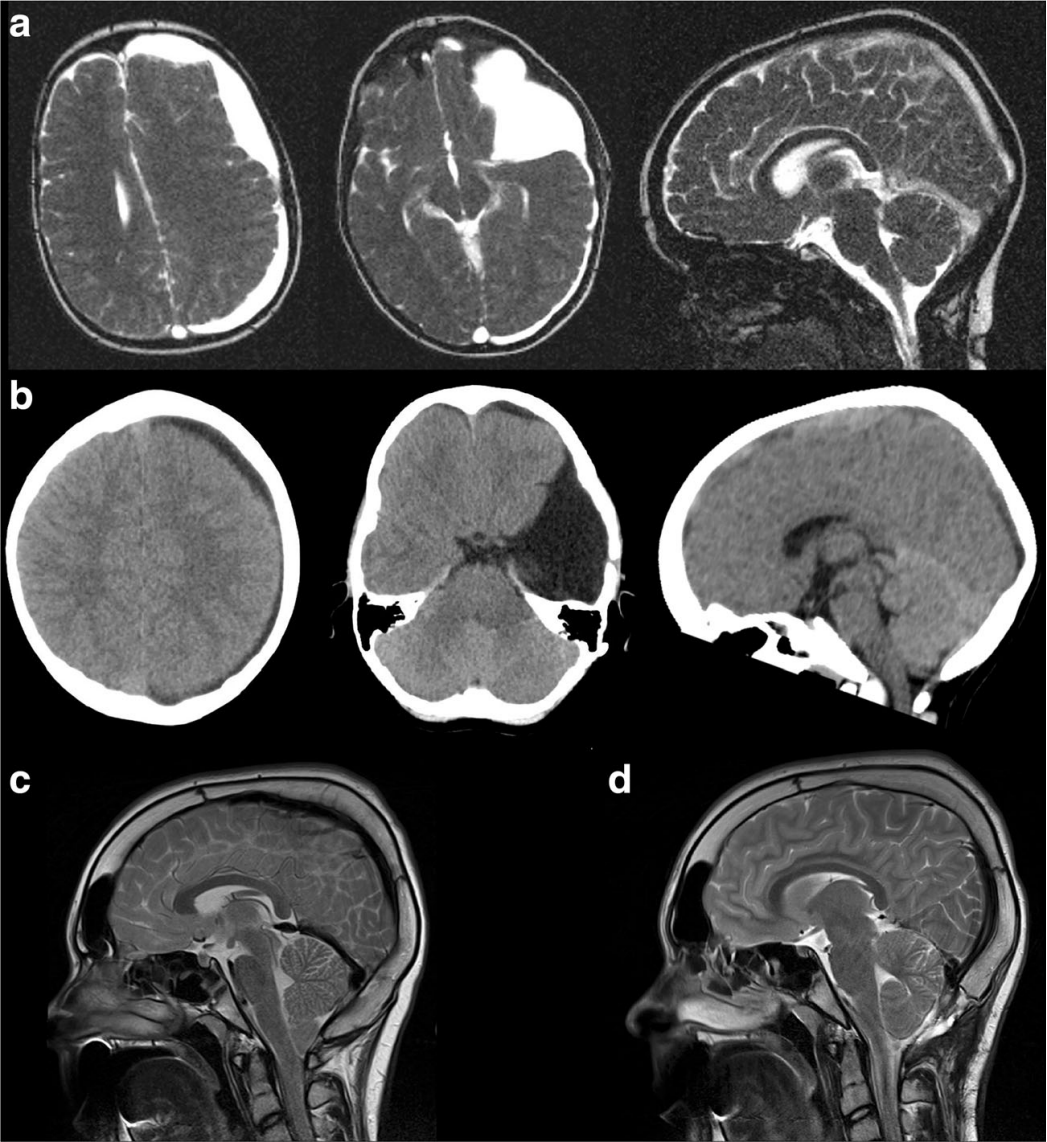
CT and MRI scans of a 3-year-old boy with a bleeding in a left frontotemporal arachnoid cyst, treated with a craniotomy with fenestration and marsupialization of the cyst. A large left-sided subdural hygroma developed at the location of the former arachnoid cyst (a) after which a subduroperitoneal drain was placed with good result (b). At the age of 14 years, he developed an acquired Chiari I malformation due to cranial vault thickening (c), which was treated with a standardized C0 augmentation, C1 laminectomy, and a dural patch, followed by thinning of the occipital planum. The postoperative MRI shows an adequate decompression with enlargement of the posterior fossa (d)
